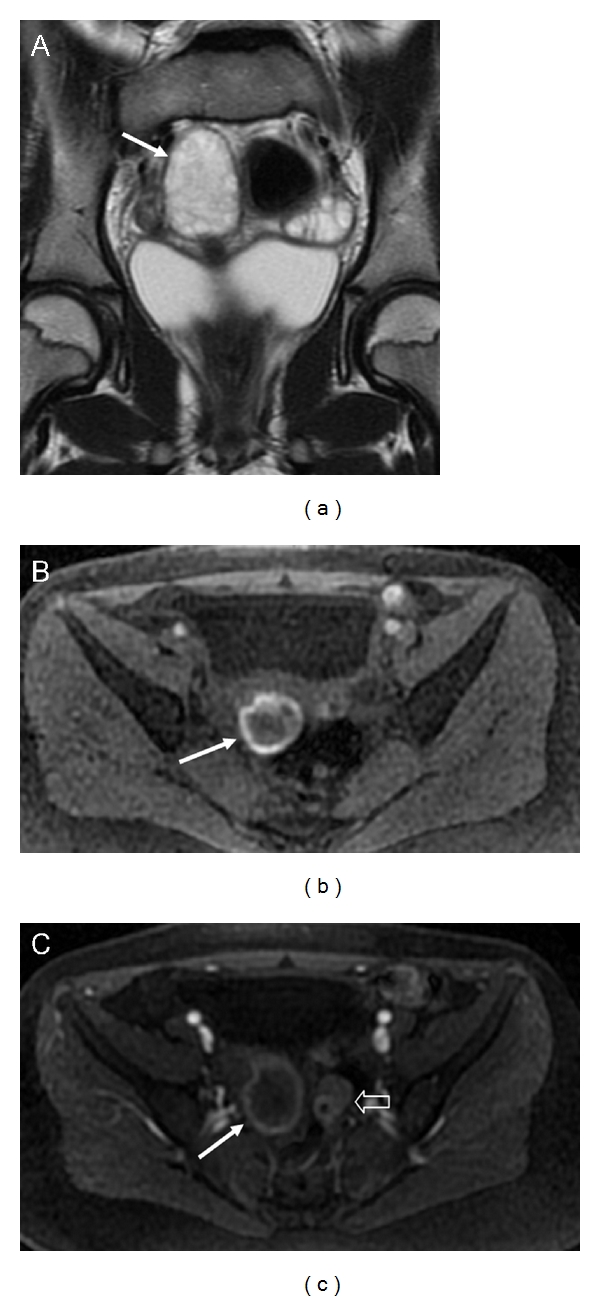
Torsion of right ovary. Coronal T2-weighted image (a) shows an enlarged right ovary with numerous small peripheral cysts representing displaced follicles (arrow). The right ovary displays a peripheral annular hyperintensity on fat suppressed T1-weighted image which is indicative of hemorrhagic infarction (b, arrow). 3D fat-saturated T1-weighted Spoiled Gradient Echo image demonstrate the lack of enhancement of ovarian parenchyma (c, white arrow). Note: normal left ovary with small normal follicle (c, void arrow).
